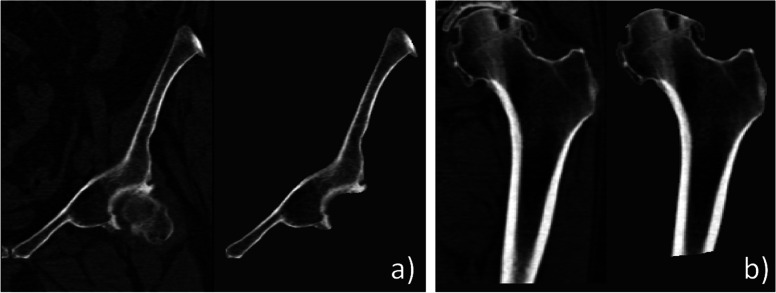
Example of reconstructed intensities after encoding with Bernstein polynomials of degree 3 for a hipbone (a) and proximal femur (b). For each bone, the left subfigure corresponds to the computed tomography image, while the right is the reconstructed image using the encoded intensities
